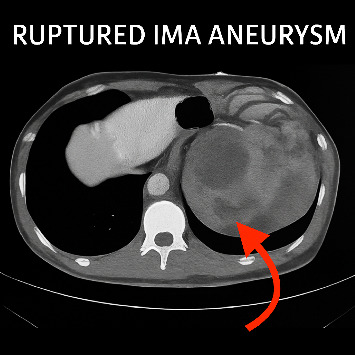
CT scan revealing ruptured IMA aneurysm with retroperitoneal hematoma (red arrow).
